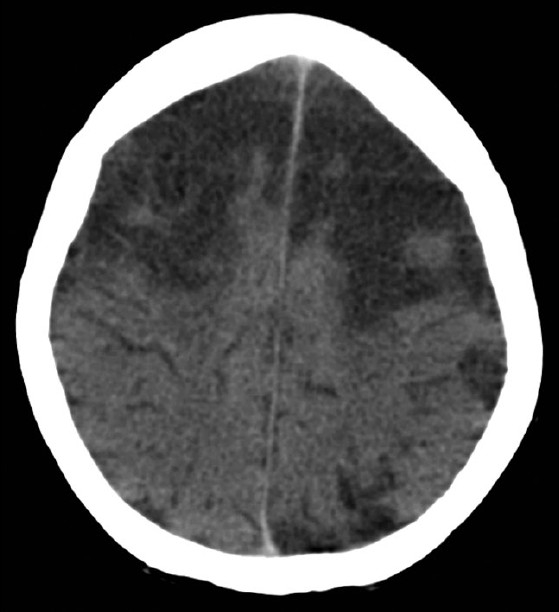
CT brain with contrast, day 6 in ICU, deteriorating neurologic status
